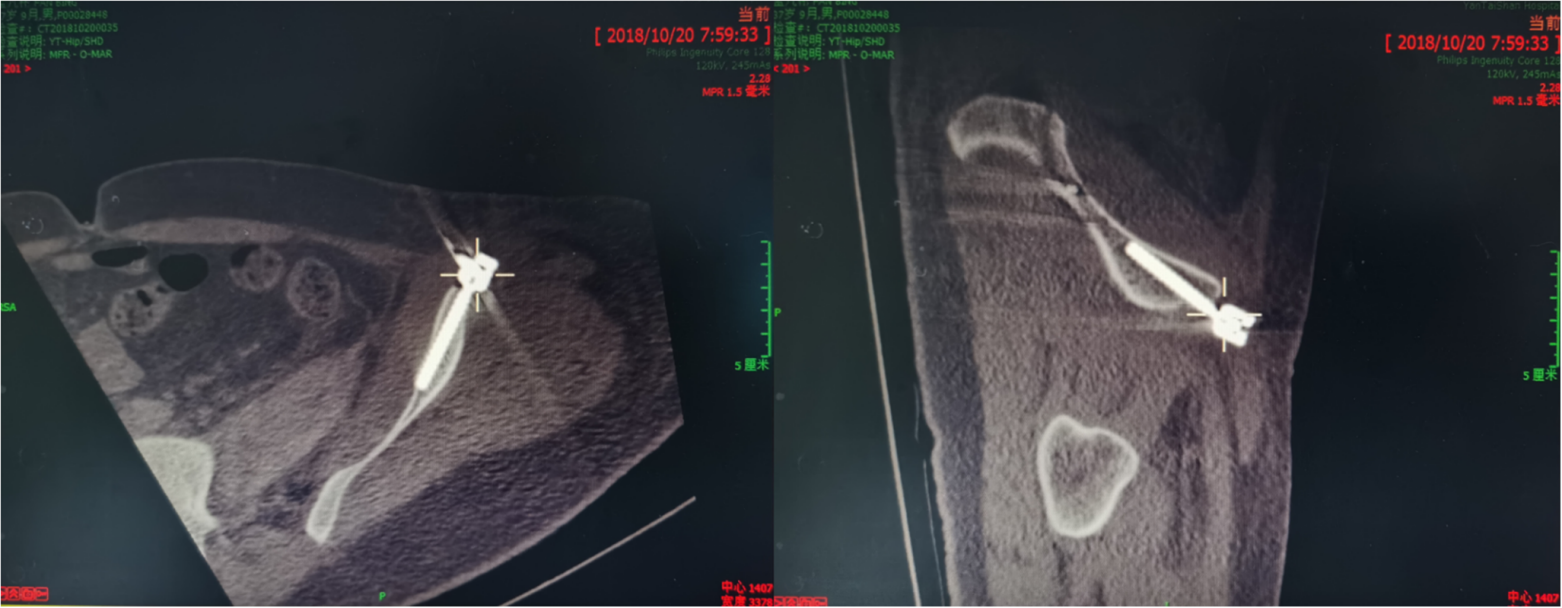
Post-operative CT scan reconstruction of the INFIX screws showed that the iliac cortex could be perforated if a longer screw was selected
